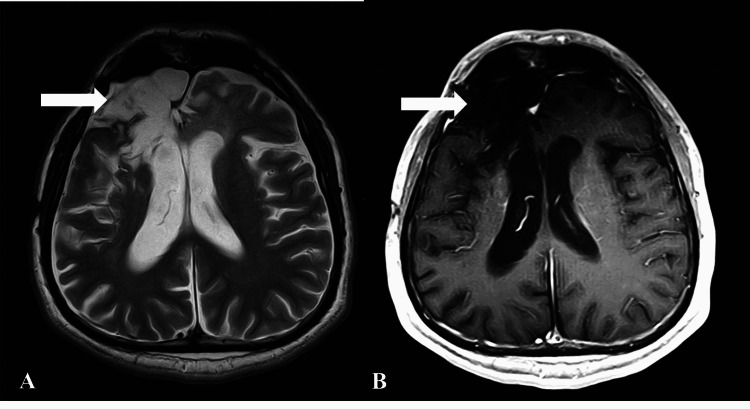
Follow-up magnetic resonance images of the brain. Postoperative and posttreatment scans after one year. (A) Axial T2-weighted sequence shows encephalomalacia at the site of surgery (arrow). (B) No significant residual disease is seen on the axial post-contrast T1-weighted image (arrow).
